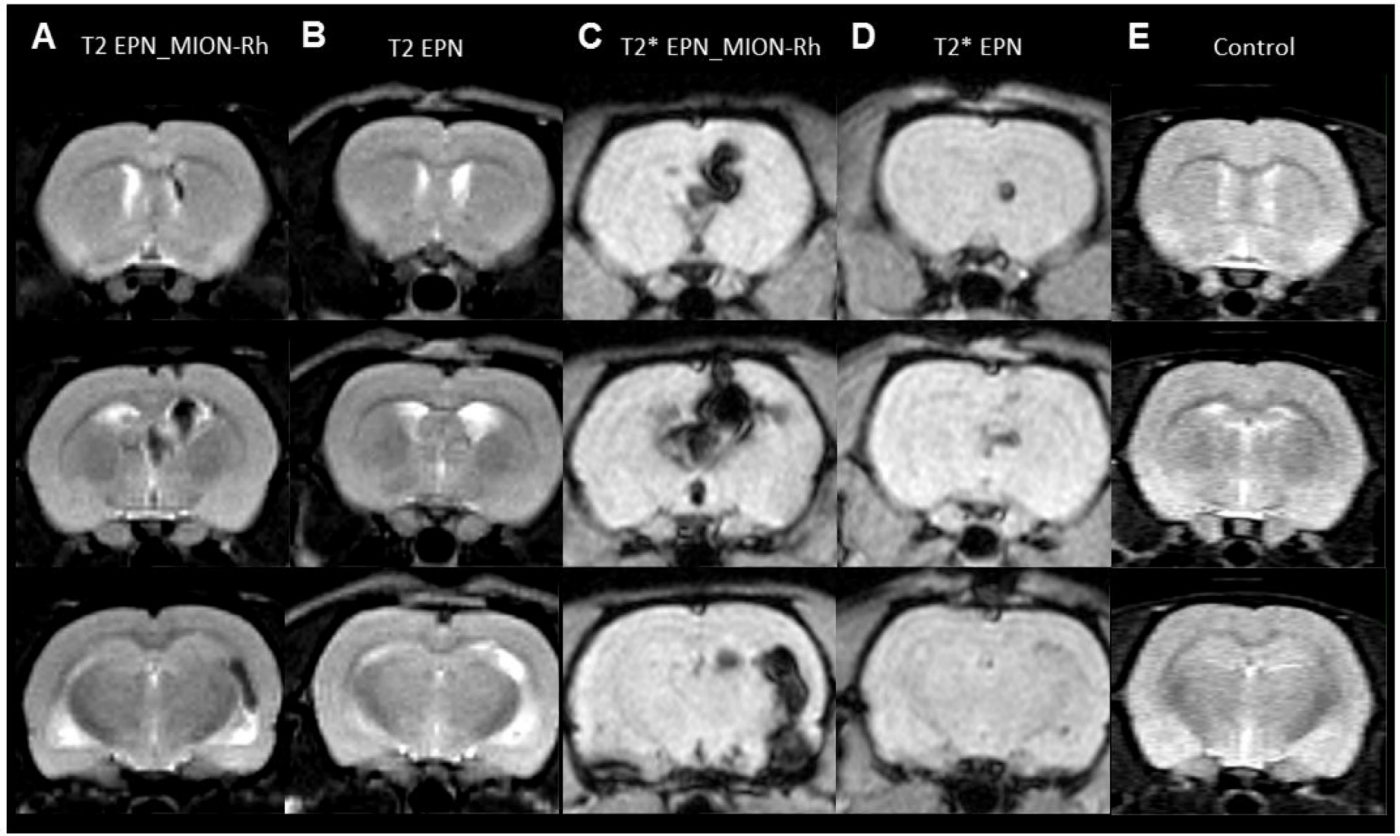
(A–E) MRI monitoring of *in vivo* EPNs. (A, B, E) T_2_-weighted images. (C, D) T_2_^*^-weighted images. (A, C) Detection of MION-Rh labeled EPN cells. (B, D) Detection of unlabeled EPN cells. (E) Control without any EPN cell transplantation.
